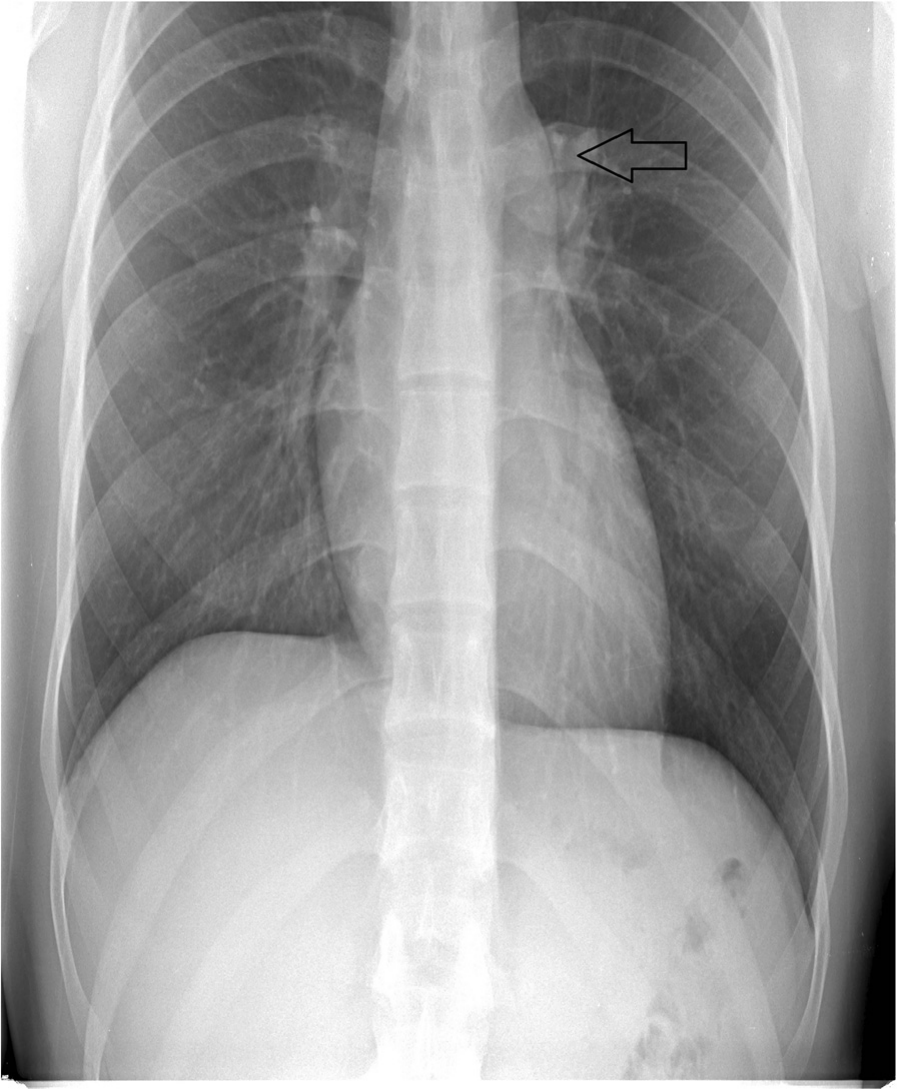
Chest X-ray, showing enlargement of the main pulmonary artery.
